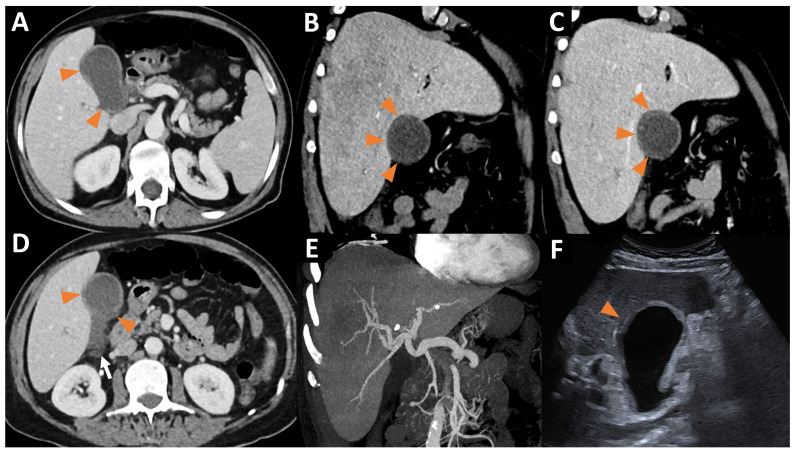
(A) Axial CT at the portal phase showing segmental enhancement defects of the gallbladder wall (orange arrowheads). (B,C) Oblique coronal CT at the arterial (B) and portal phase (C) showing a segmental enhancement defect of the hepatic side of the gallbladder wall (orange arrowheads). Again, we found that the heterogeneous hepatic enhancement was linked to the arteriovenous shunt between the left branch of the hepatic artery and the left hepatic vein at the arterial phase (B). (D) Axial CT at the portal phase showing thickening of the gallbladder wall with segmental enhancement defects of the gallbladder wall (orange arrowheads) and a perivesicular fluid effusion (white arrow). (E) Coronal CT at the arterial phase with MIP 30 mm, showing a significant enlargement of the hepatic artery. (F) Mode B ultrasound showing thickening of the gallbladder wall, with a hypoechoic area of the hepatic side of the gallbladder wall (orange arrowhead), corresponding to the areas of segmental enhancement defects visualized on CT. Ultrasound revealed no gallstones in the gallbladder.
